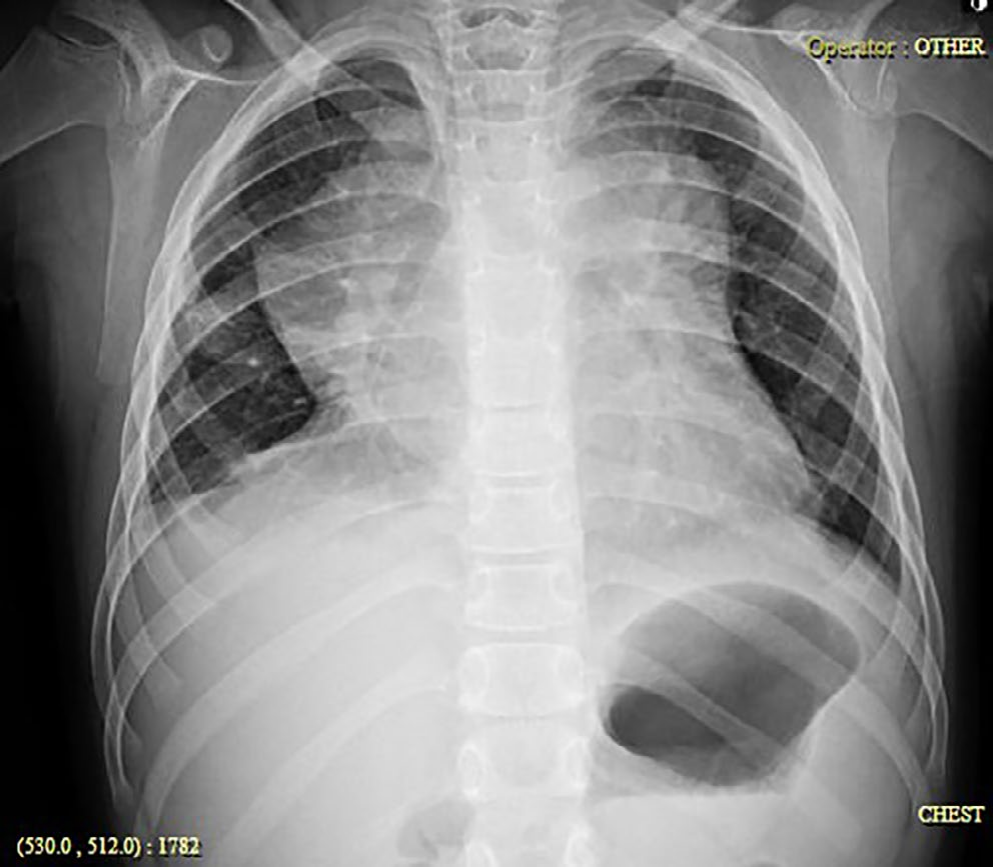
Patient's chest radiograph demonstrating a mediastinal widening, suggestive of mediastinal mass
